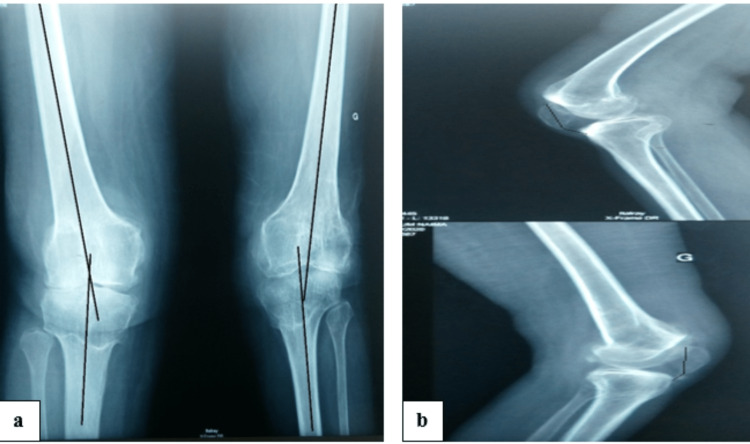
Frontal (a) and lateral (b) preoperative X-ray showing severe valgus knee with patella baja for both knees.
